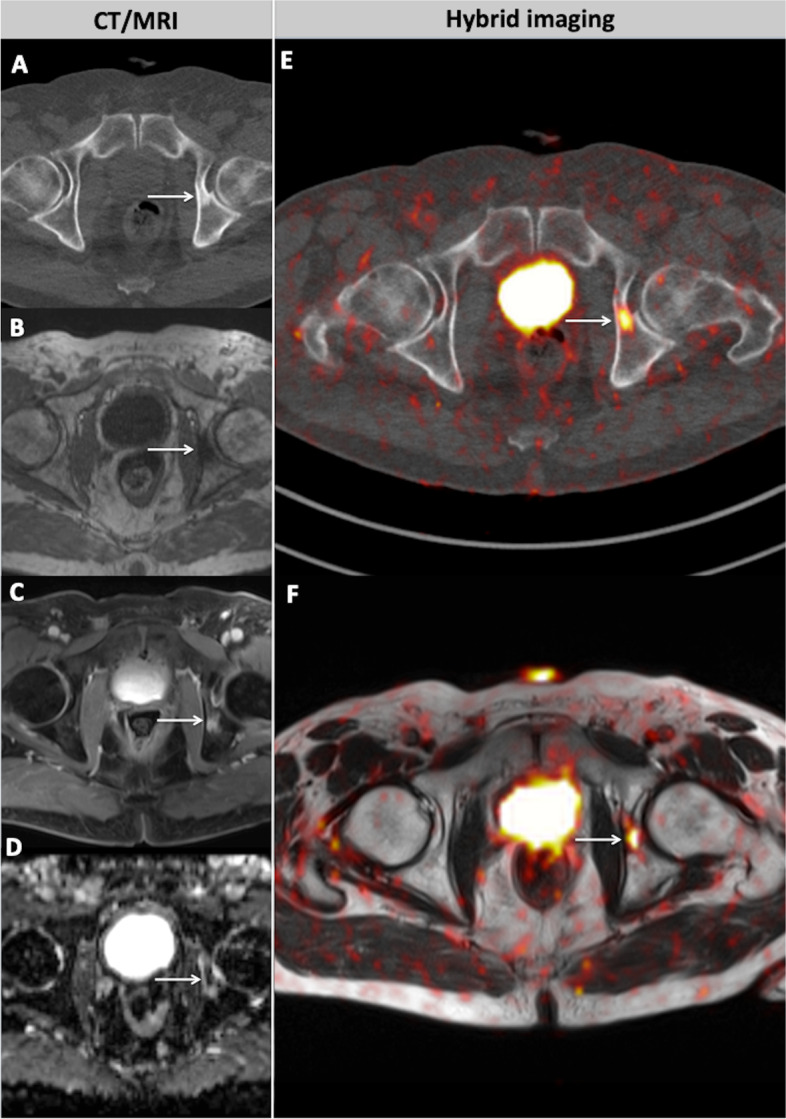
A 74-year old patient with biochemical recurrence of PCa after radical prostatectomy (PSA = 1.68 ng/ml). The patient showed a sclerotic lesion in the left acetabulum as a sign of an osseous metastasis in CT (A, arrow). In MRI, correspondingly, the lesion appears hypointense in T1w (B, arrow) with contrast enhancement (C, arrow) and signal decrease in ADC map of the DWI (D, arrow). Respectively, both hybrid imaging modalities PET-CT (E, arrow) and PET-MRI (F, arrow) depict the osseous lesion as a focus of markedly increased [^68^Ga]PSMA-11 uptake, highly suggestive of metastasis
